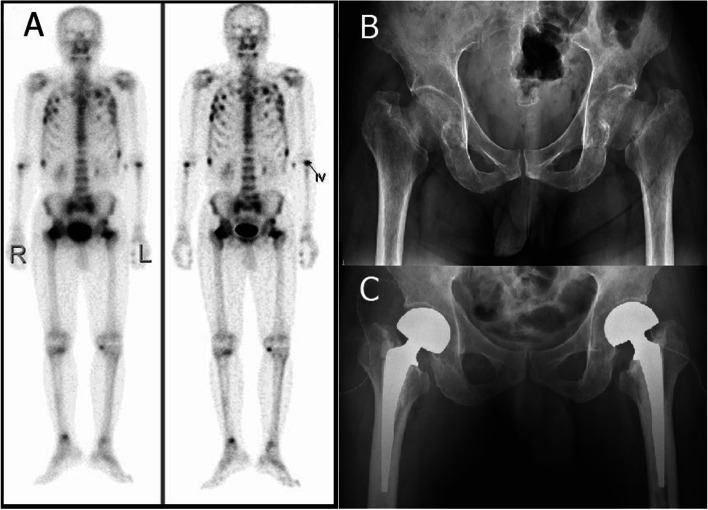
A Whole body bone scan: multiple hot spots of uptake located in the ribs, spine, sacrum ala, and bilateral hips. B Plain radiographs of the pelvis: bilateral femoral neck insufficiency fractures with displacement. C Post-operative plain radiographs of the pelvis
